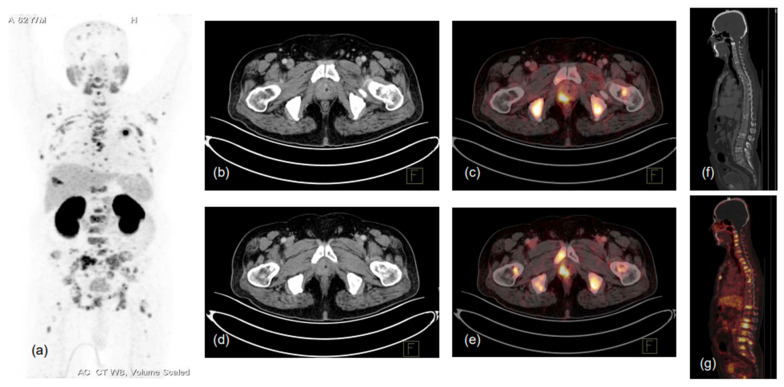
62-year-old male, presented with lower urinary tract symptoms (LUTS) and hematuria. USG of the whole abdomen showed prostatomegaly, and serum PSA was 480.43 ng/mL. TRUS-guided prostate biopsy showed infiltrating acinar adenocarcinoma, Gleason score 5 + 4 = 9. Patient was referred for PSMA PET-CT scan due to high-risk features. ^68^Ga-PSMA-11 PET-CT scan showed prostatomegaly with PSMA expressing heterogeneously, enhancing lesions in the right peripheral zone involving mid-gland and apical regions (SUVmax 17.0), with low-grade PSMA expressing a few tiny right internal iliac lymph nodes (SUVmax 2.8), and PSMA expressing multiple predominantly sclerotic and marrow lesions involving almost entire visualised skeleton (reference SUVmax 26.2 in sacrum). (a)-MIP image, (b–g)-CT and fused PET-CT trans-axial and sagittal images.
